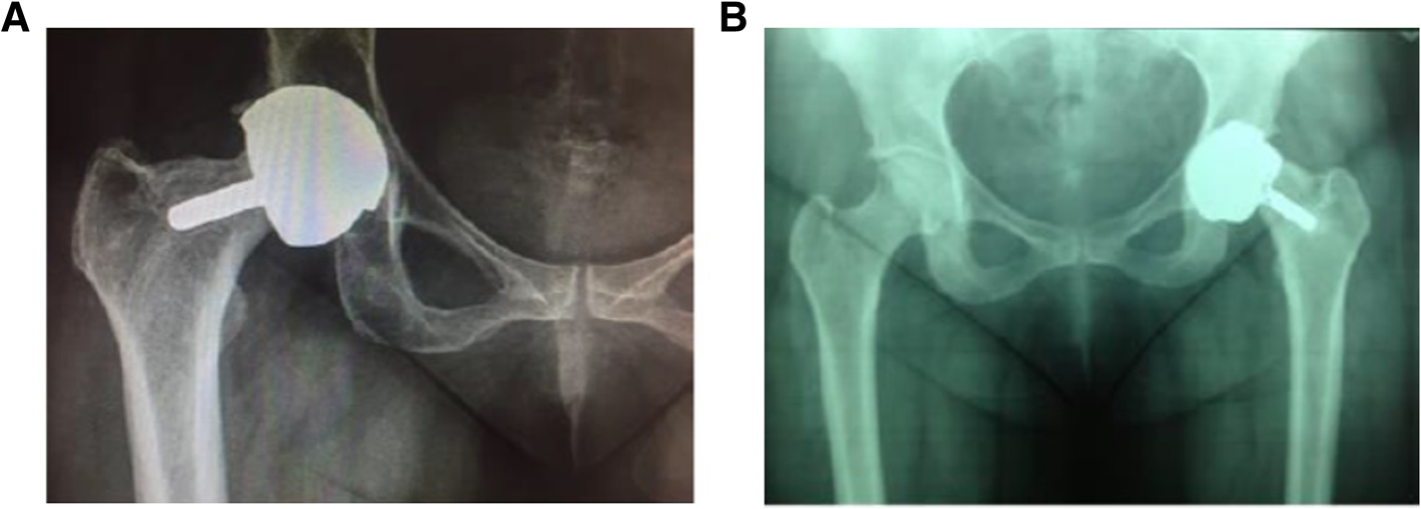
Radiographic images showing evidence of neck thinning in two patients: case 1 (a) and case 2 (b)
